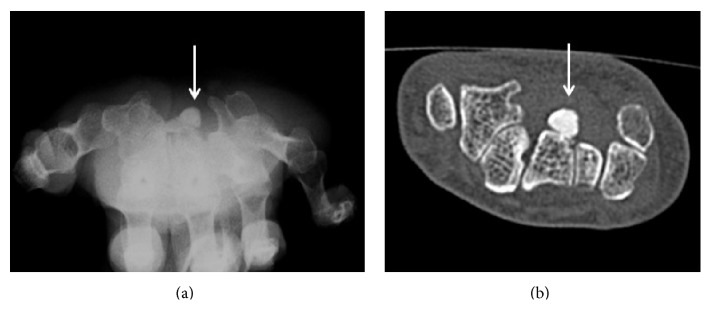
A plain radiograph showing a calcified mass on the volar surface of the wrist joint (a). A computed tomogram showing a lesion that has a high-density mass (b).
